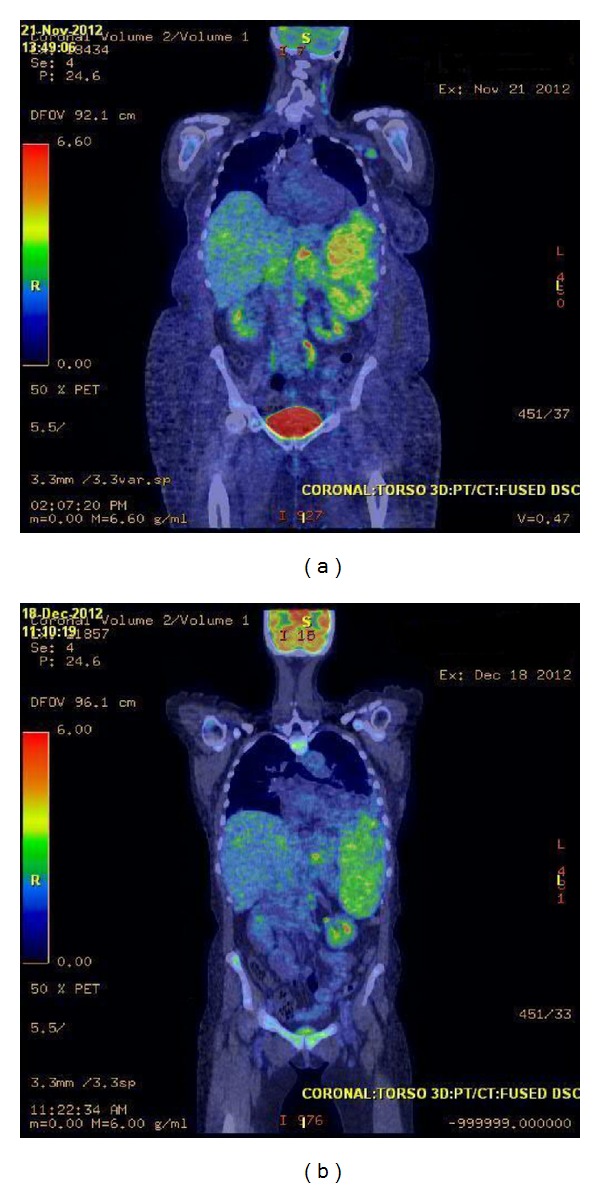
(a) PET/CT scan prior to chemotherapy. (b) PET/CT scan after 2 cycles of chemotherapy.
